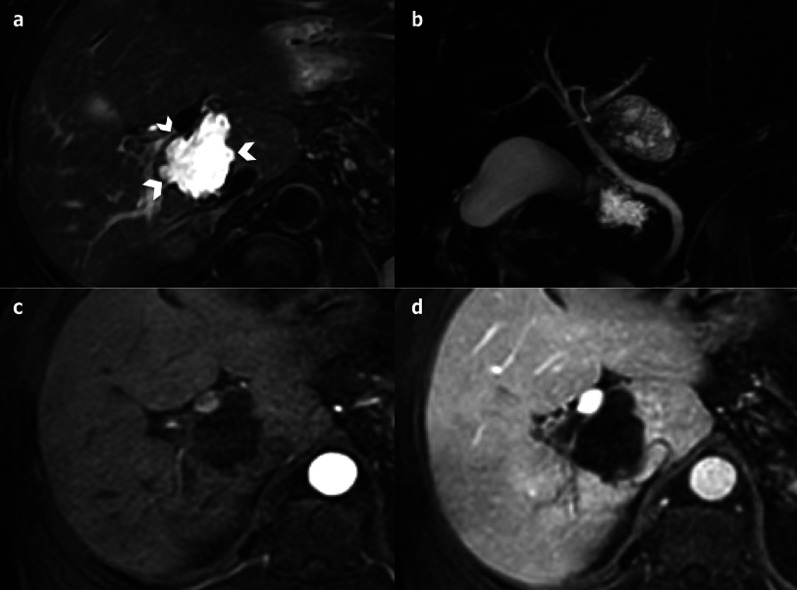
Complicated hydatid cyst in a 50-year-old female. Imaging displays a cyst very close to the biliary tract with lobulated margins, showing a loss of intralesional pressure, most probably resulting from a complication of the biliary connection (not found on magnetic resonance cholangiopancreatography). a Axial T2-weighted magnetic resonance imaging. b Coronal 3D magnetic resonance cholangiopancreatography. c and d Axial T1-fat-sat-weighted imaging without and with contrast on portal venous phase
